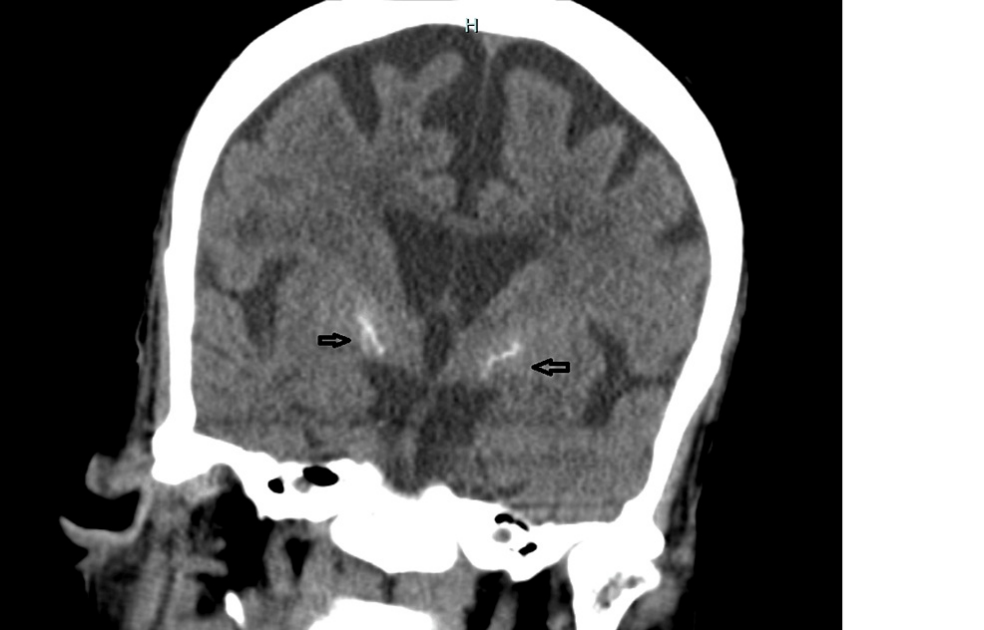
CT scan of the head without contrast showing calcifications within her basal ganglia and generalized atrophy with involutional changes.
